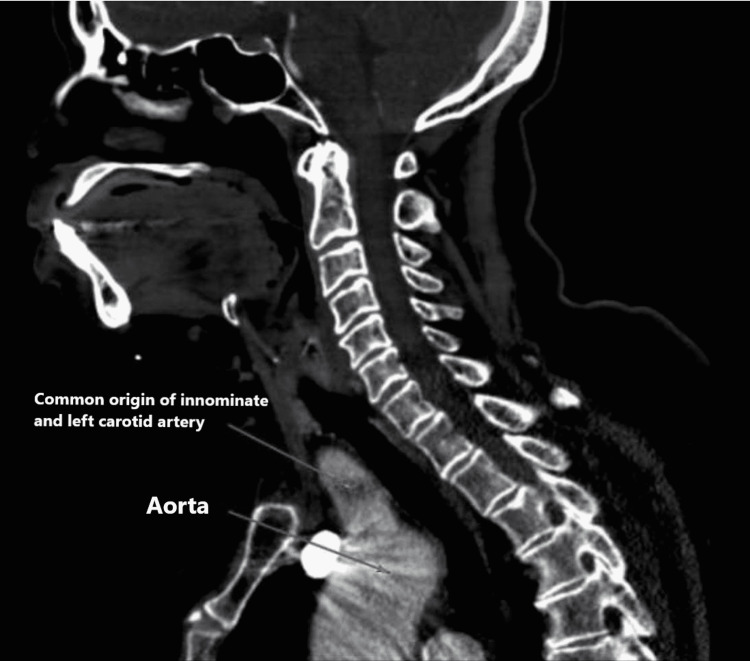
Sagittal view of CT chest shows the common origin of innominate and left carotid arteries.
